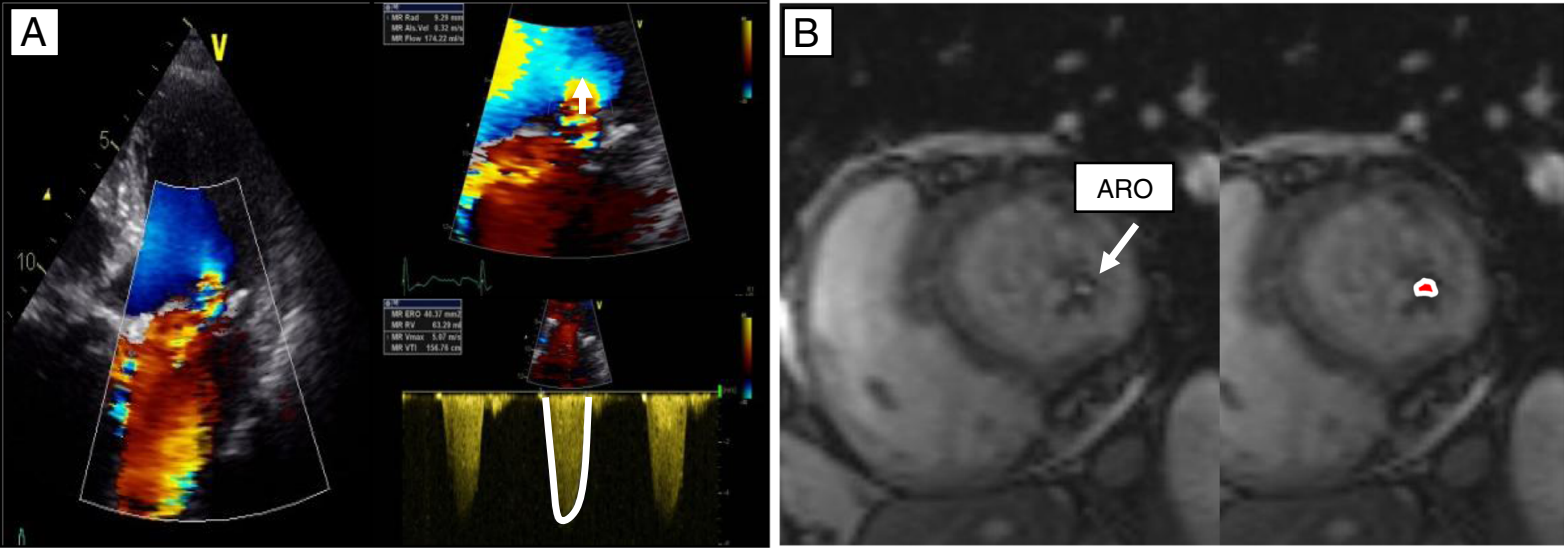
Measurement of the regurgitant orifice by 2D TTE and CMR. A. Acquisition of PISA radius and continuous wave Doppler of the MR jet allows calculation of the effective regurgitant orifice. The PISA radius is measured at mid-systole using the first aliasing with a reduced Nyquist limit (15–40 m/s). B. The anatomic regurgitant orifice can be measured by planimetry on a slice parallel to the valvular plane obtained by cardiovascular magnetic resonance.
